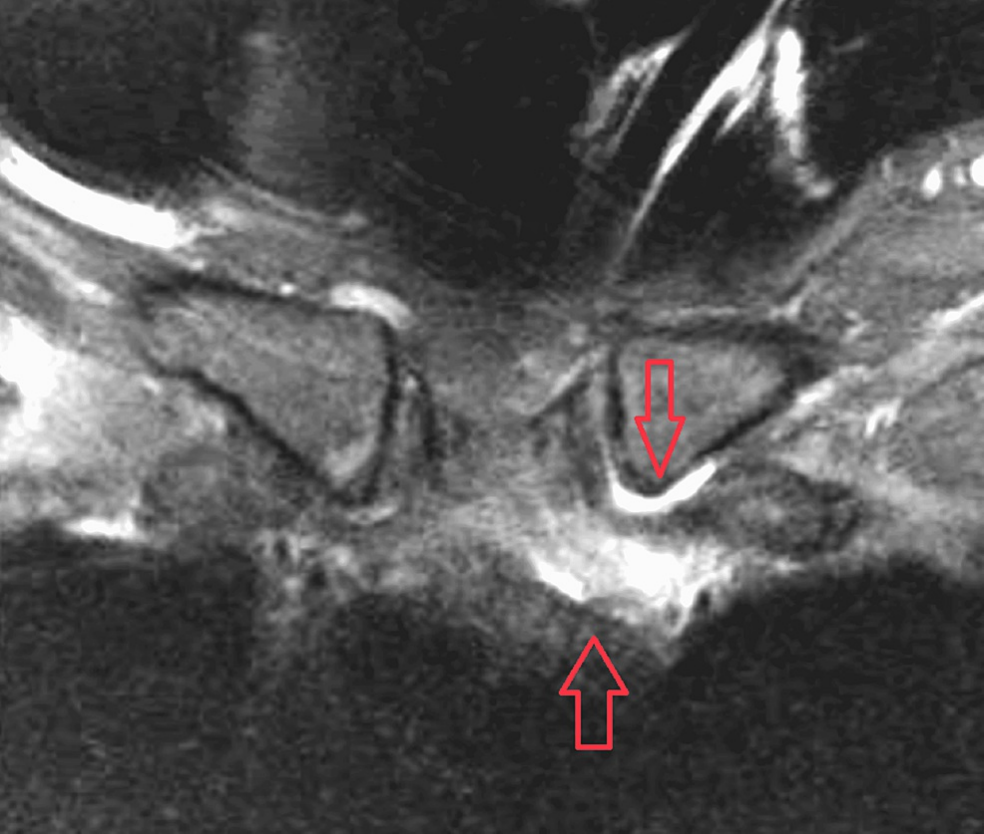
Magnetic resonance imaging of the chest showing osteomyelitis in the manubrium (arrow) with associated phlegmonous change and evidence of septic arthritis (arrow) in the left sternoclavicular joint
